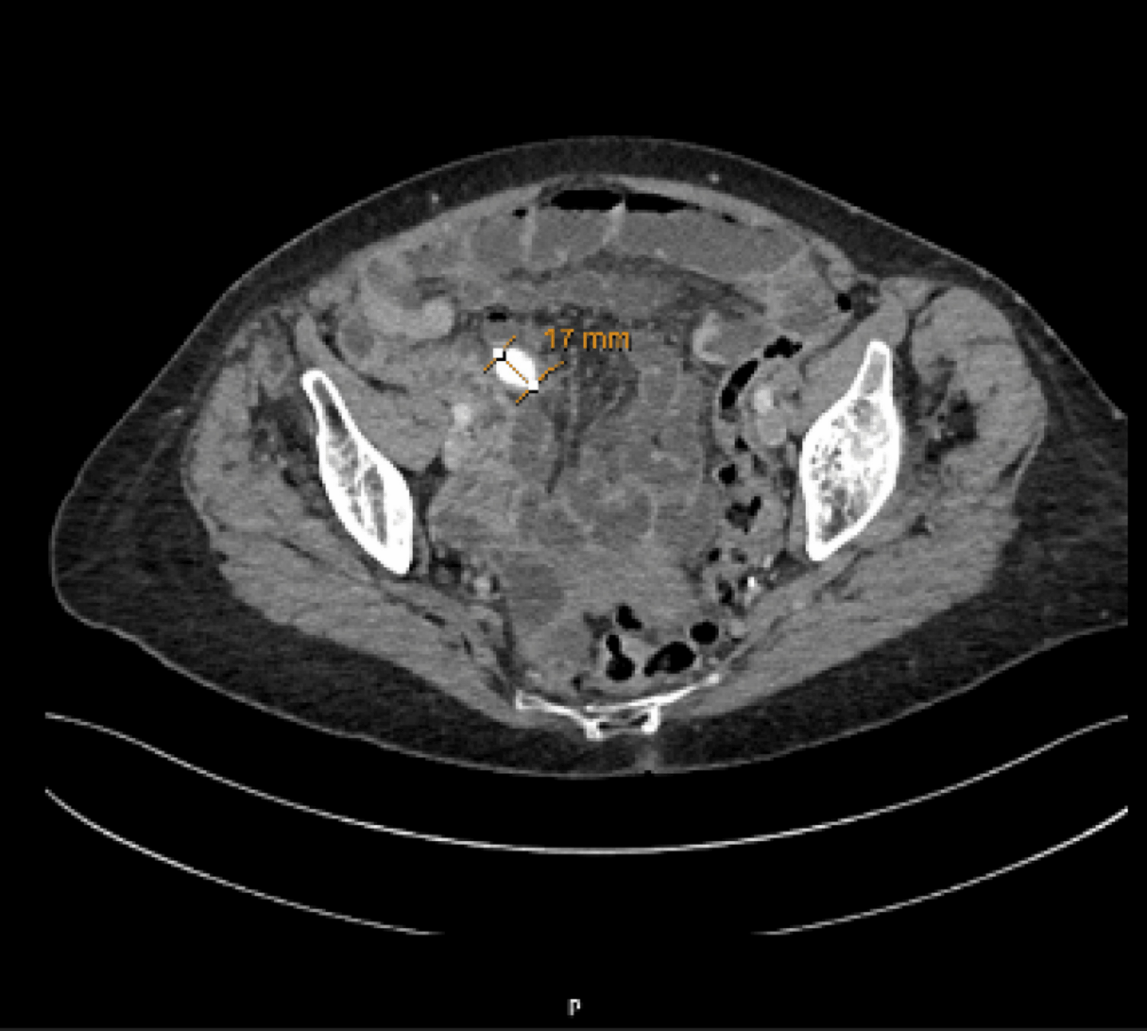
Axial CT Axial CT demonstrates a rounded, well-defined mass measuring 17 mm in diameter within the intestinal lumen.
